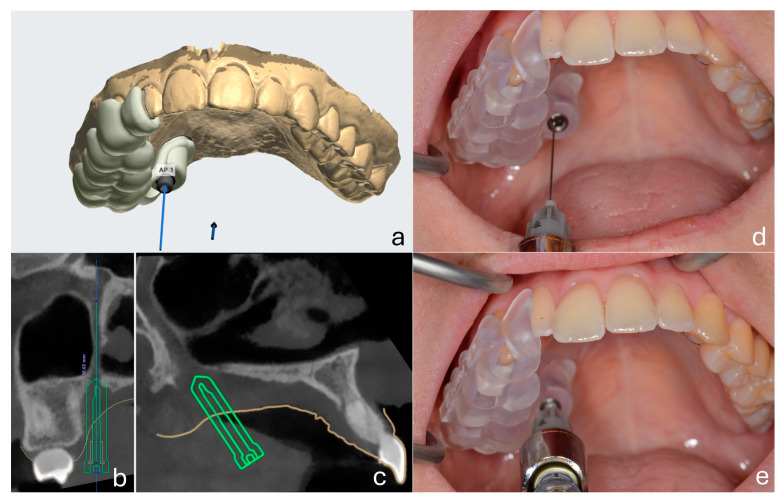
Computer-guided maxillary nerve block via the greater palatine canal using a customized surgical guide. (a) Virtual planning of the guide and insertion trajectory on maxillary digital cast. (b,c) CBCT sections showing the planned path of needle insertion into the pterygopalatine canal in relation to surrounding anatomy. (d,e) Clinical application of the 3D-printed guide with the needle inserted through the metallic sleeve during local anesthetic administration.
